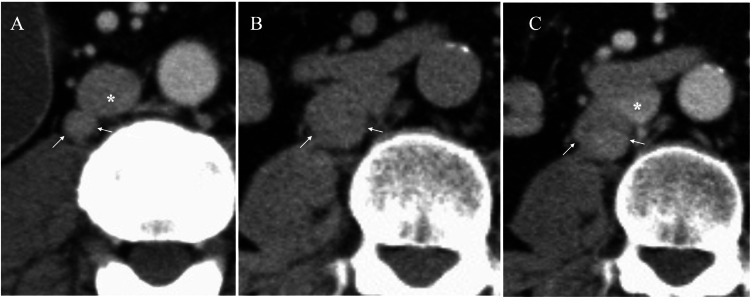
Computed tomography (CT) findings A. CT showed a mass (arrows) located just adjacent to the inferior vena cava (asterisk). B. CT showed enlargement of the mass (arrows). C. CT showed nominal enhancement of the tumor (arrows) with indistinct borders with the inferior vena cava (asterisk).
